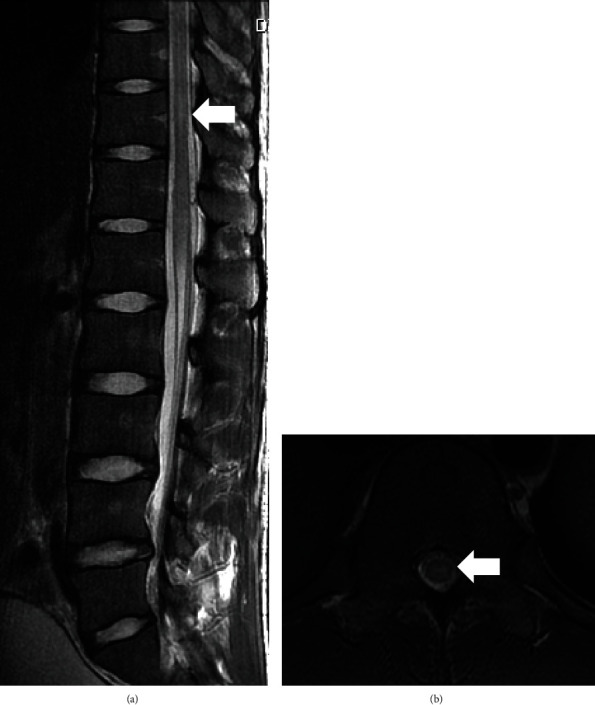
(a) Sagittal MRI T2-weighted imaging (T2WI) of the spine at the time of admission showed a hyperintense lesion in the spinal cord at the Th11 level of the lower spine (arrow). (b) Axial MRI T2-weighted imaging (T2WI) of the Th11 level of the spine at the time of admission showed a hyperintense lesion in the spinal cord (arrow).
